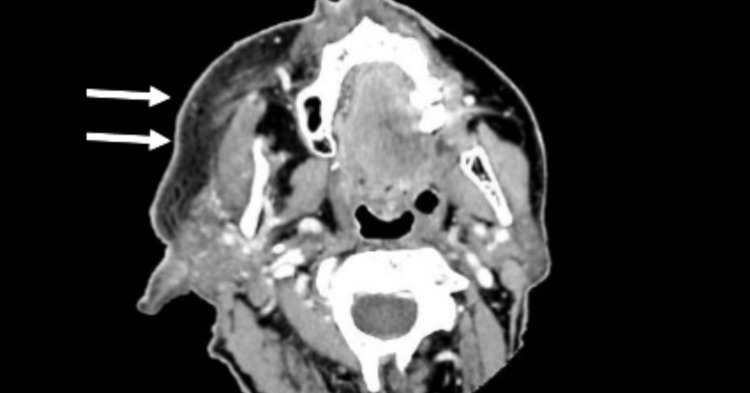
Maxillofacial CT scan: asymmetric subcutaneous edema and mild inflammation throughout the right side of the face (white arrows). No abscess.
